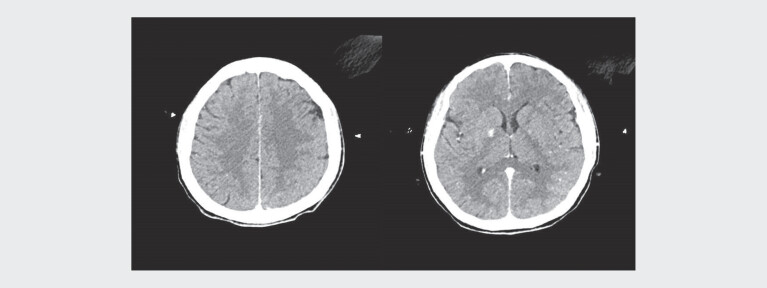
Cranial computed tomography manifestations 15 hours
